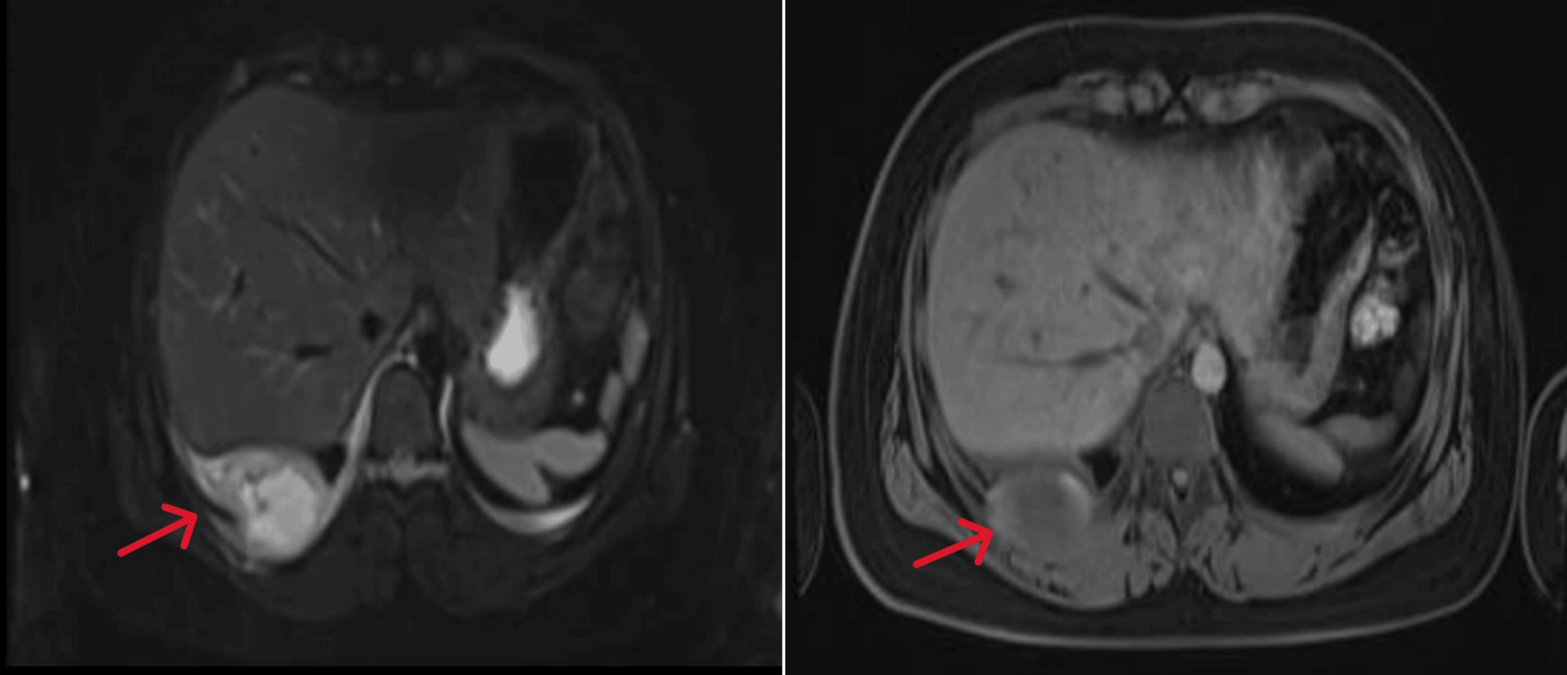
Extraosseous Ewing's sarcoma (EES) magnetic resonance imaging (MRI) of the right chest wall non-enhanced examination. A. Axial T2-weighted imaging fat suppression (T2WI-FS) showed high and slightly low signal; B. Axial T1-weighted imaging (T1WI) showed slightly low signal.
